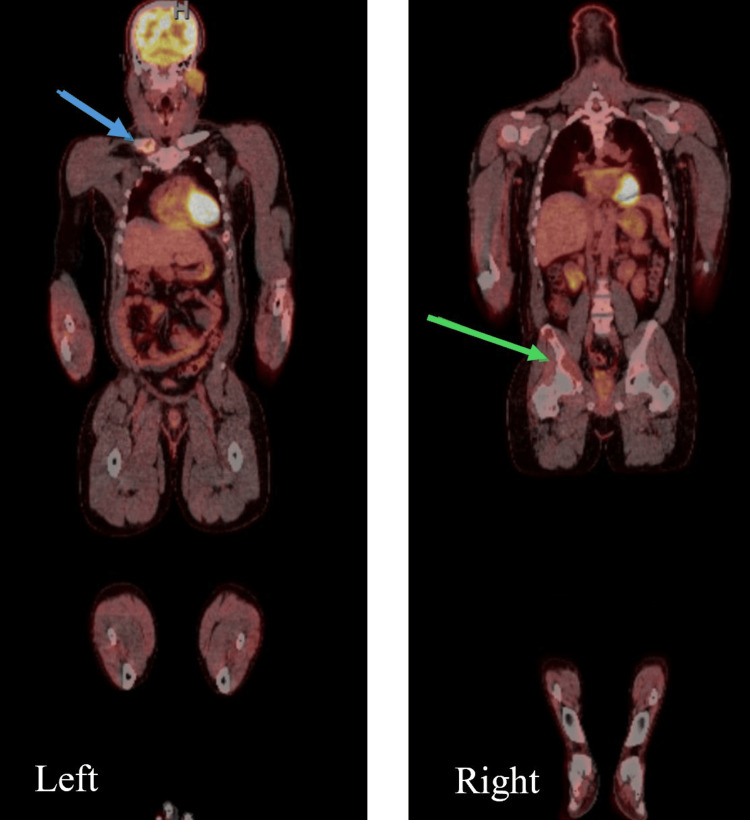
Total body PET/CT (Positron Emission Tomography / Computed Tomography) scan showing known widespread lesions with increased fluorodeoxyglucose uptake in the head of the right clavicle (left image, blue arrow) and right iliac bone and acetabulum (right image, green arrow), indicating areas with increased metabolic activity.
